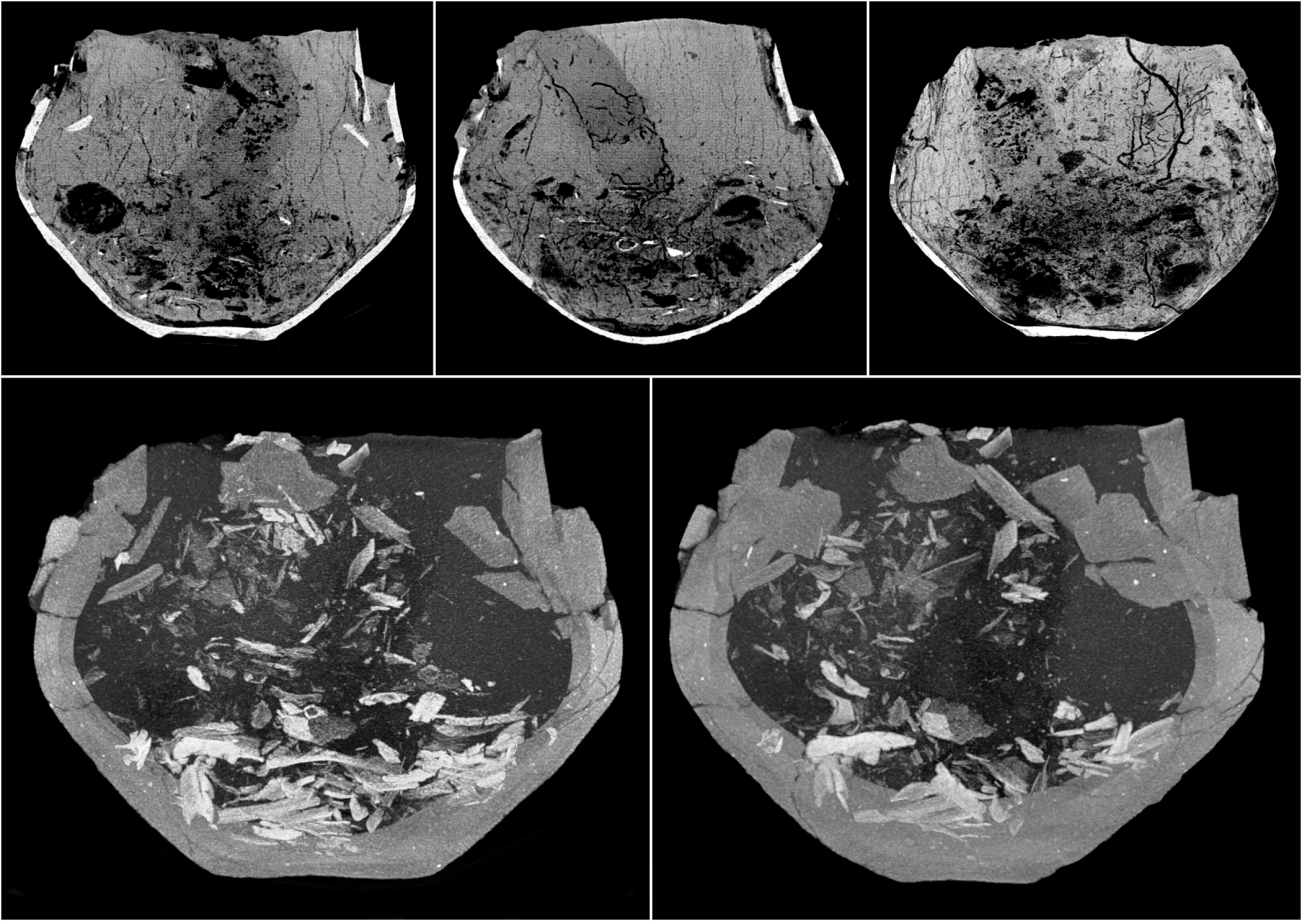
The CT imaging of the fill of the urn from grave 877. The top row shows the MinIP reconstruction of the urn fill with a visible system of burrows and ingrown plant roots. At the bottom, the MIP reconstruction of the displaced upwards and mixed burnt bones.
